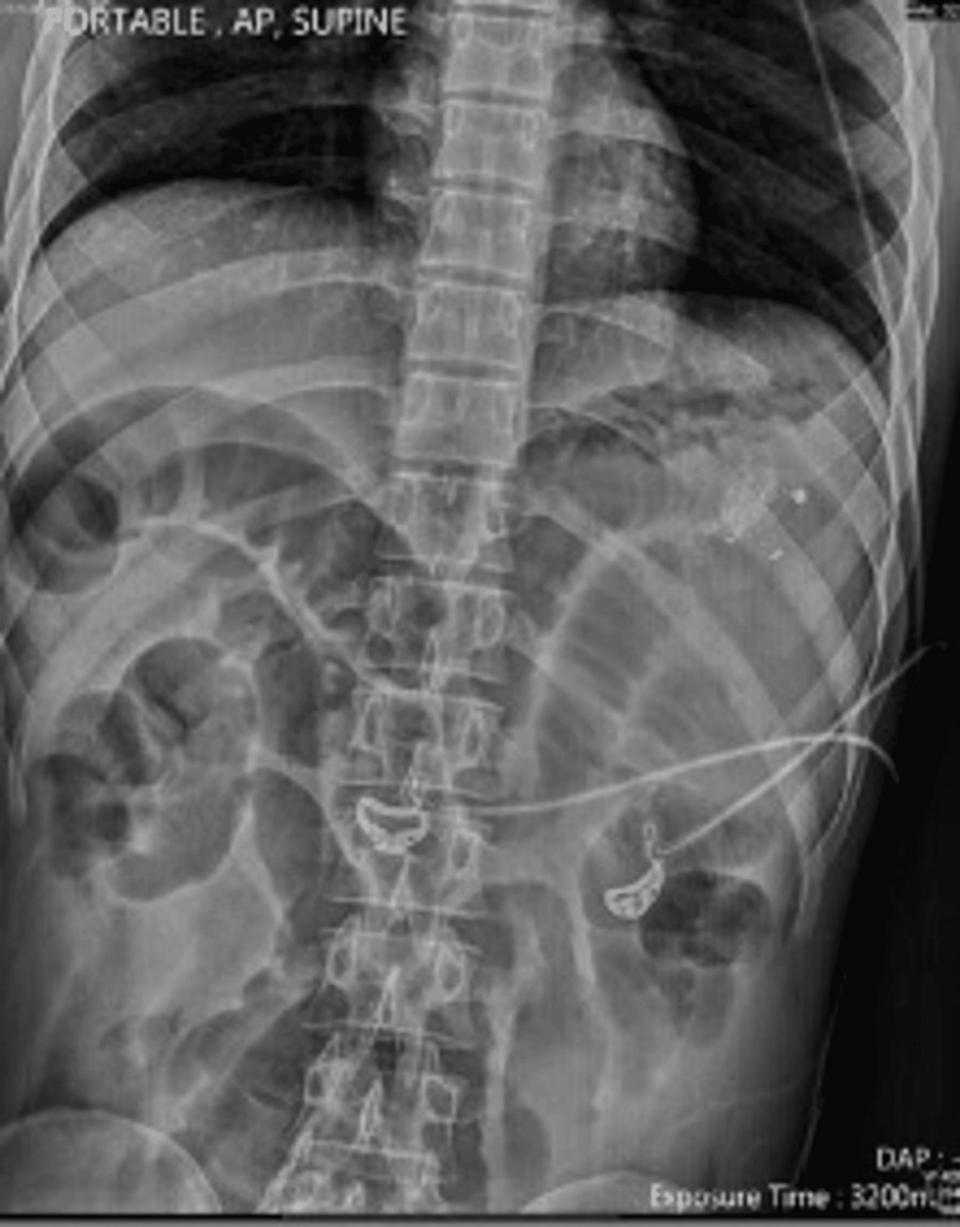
Supine X-ray. Dilated bowl loops can be noted. The patient was not able to undergo an X-ray in an erect position because he was unstable.
